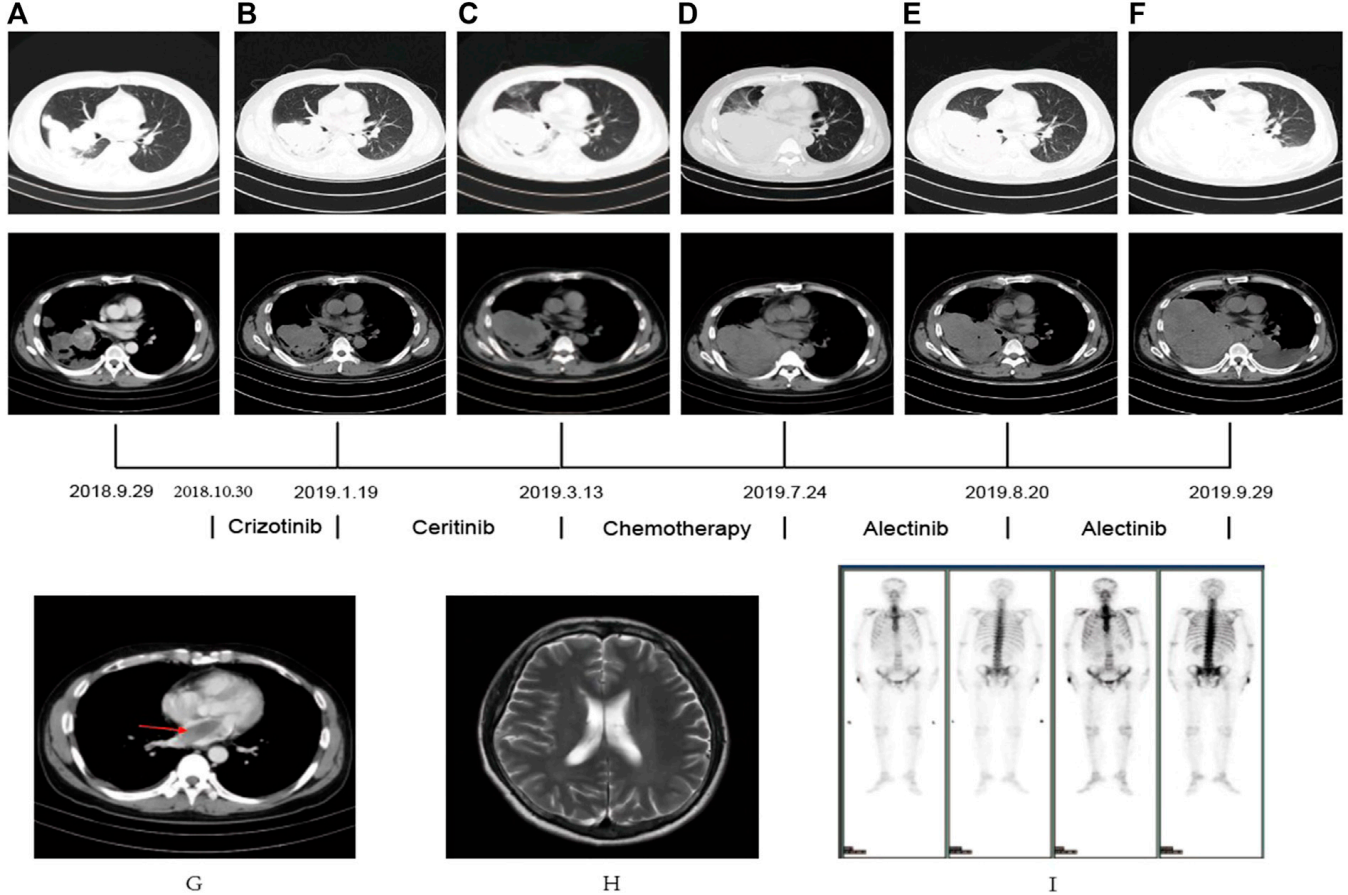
(A–F) CT scan showed the changes in the lung mass in the patient PRE- and POST-treatment (G) The CT scan revealed an atrial filling defect. (H,I) MRI and PET-CT showed no metastasis in brain and bone.
